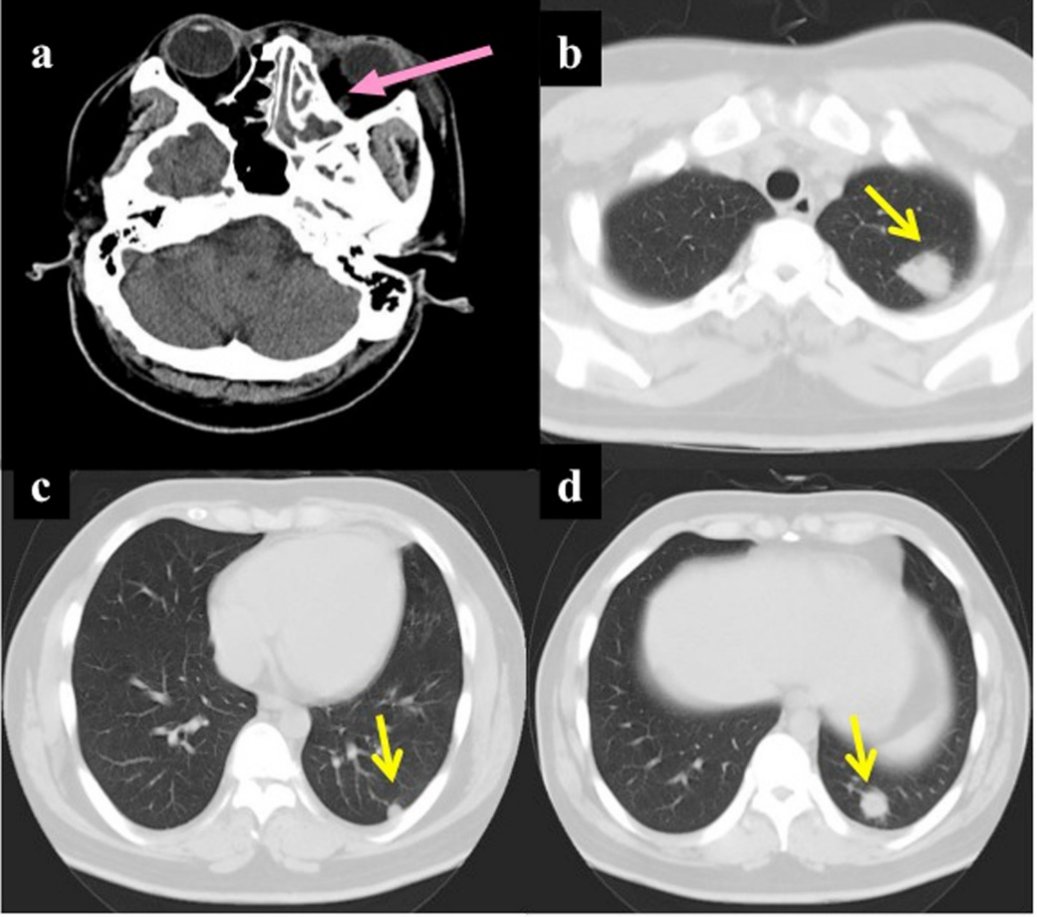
Whole body computed tomography scan before treatment. (a) The left nasal cavity was filled with soft tissue (pink arrow). (b, c, d) Multiple lung nodules were identified (yellow arrows).
